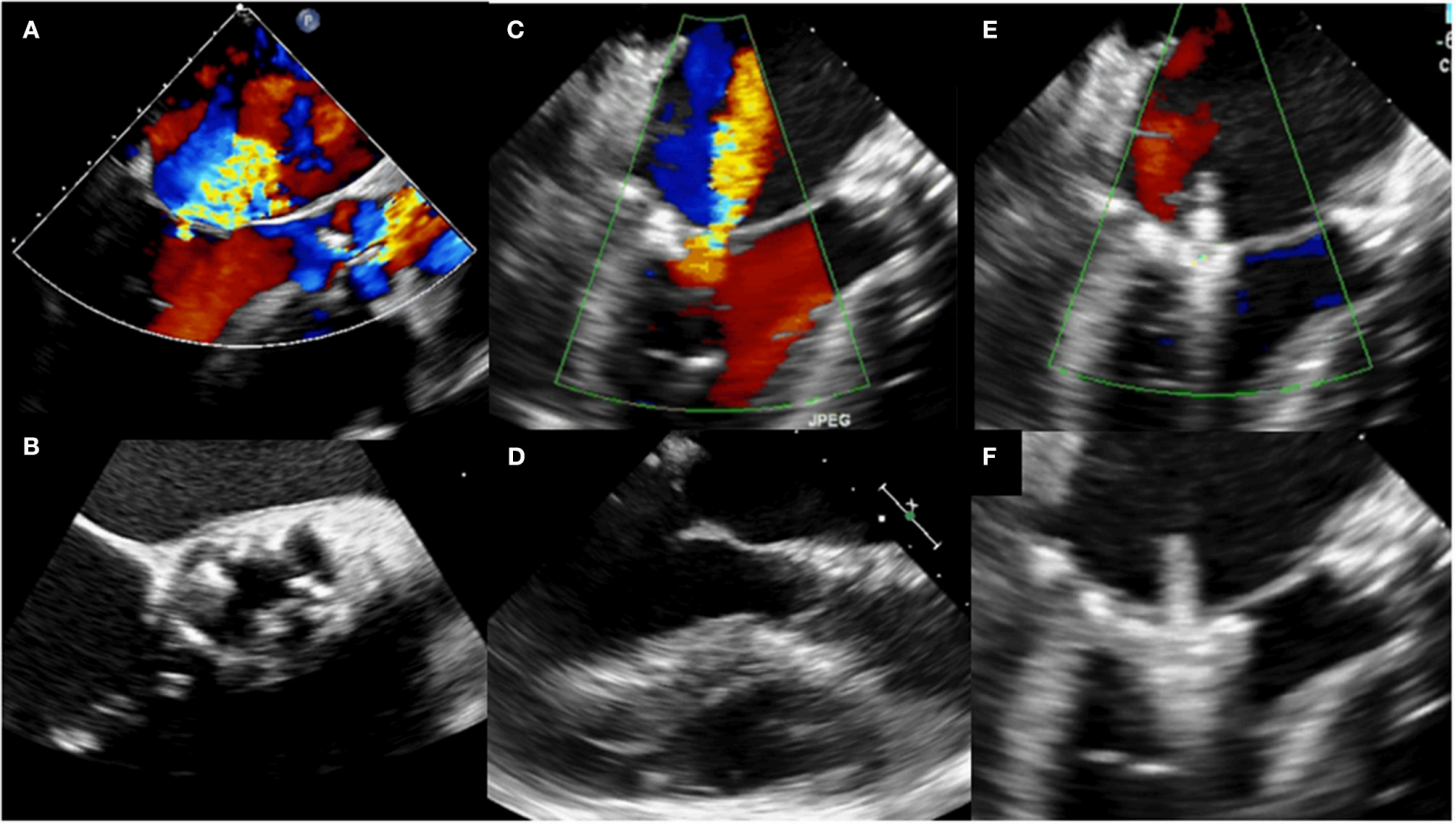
Pre- and post-procedural transesophageal echocardiography in a patient undergoing staged transcatheter aortic valve replacement (TAVR) and edge-to-edge mitral valve repair with the MitraClip® system. (A) Transesophageal color Doppler echocardiography at baseline (three-chamber view) showing severe aortic stenosis and concomitant severe mitral regurgitation. (B) Transesophageal echocardiography at baseline (aortic valve short-axis view) showing severe aortic stenosis. (C) Two-dimensional transesophageal color Doppler echocardiography (three chamber view) showing persistent severe mitral regurgitation following TAVR. (D) Two-dimensional transesophageal echocardiography (three chamber view) following TAVR. (E) Two-dimensional transesophageal color Doppler echocardiography (three-chamber view) during staged percutaneous edge-to-edge mitral valve repair with the MitraClip® system (grasping). (F) Two-dimensional transesophageal echocardiography (three-chamber view) during staged percutaneous edge-to-edge mitral valve repair with the MitraClip® system (grasping).
